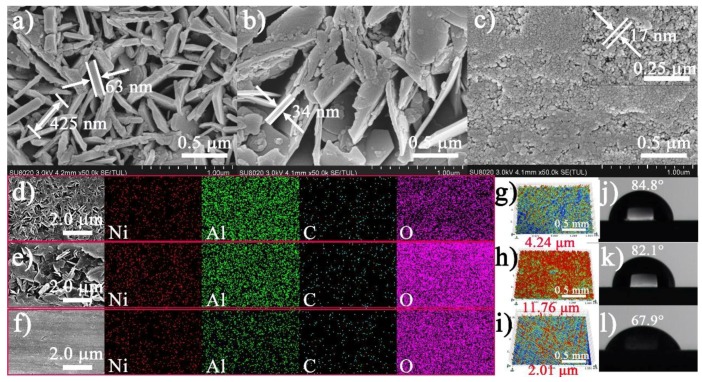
(a–c) SEM; (d–f) SEM-EDS mapping; and (g–i) 3D roughness images of the surface morphologies; and (j–l) water contact angles for (a,d,g,j) NiAl-LDH/CT, (b,e,h,k) NiAl-LDH/NT, and (c,f,i,l) NiAl-LDH/ST coatings.
